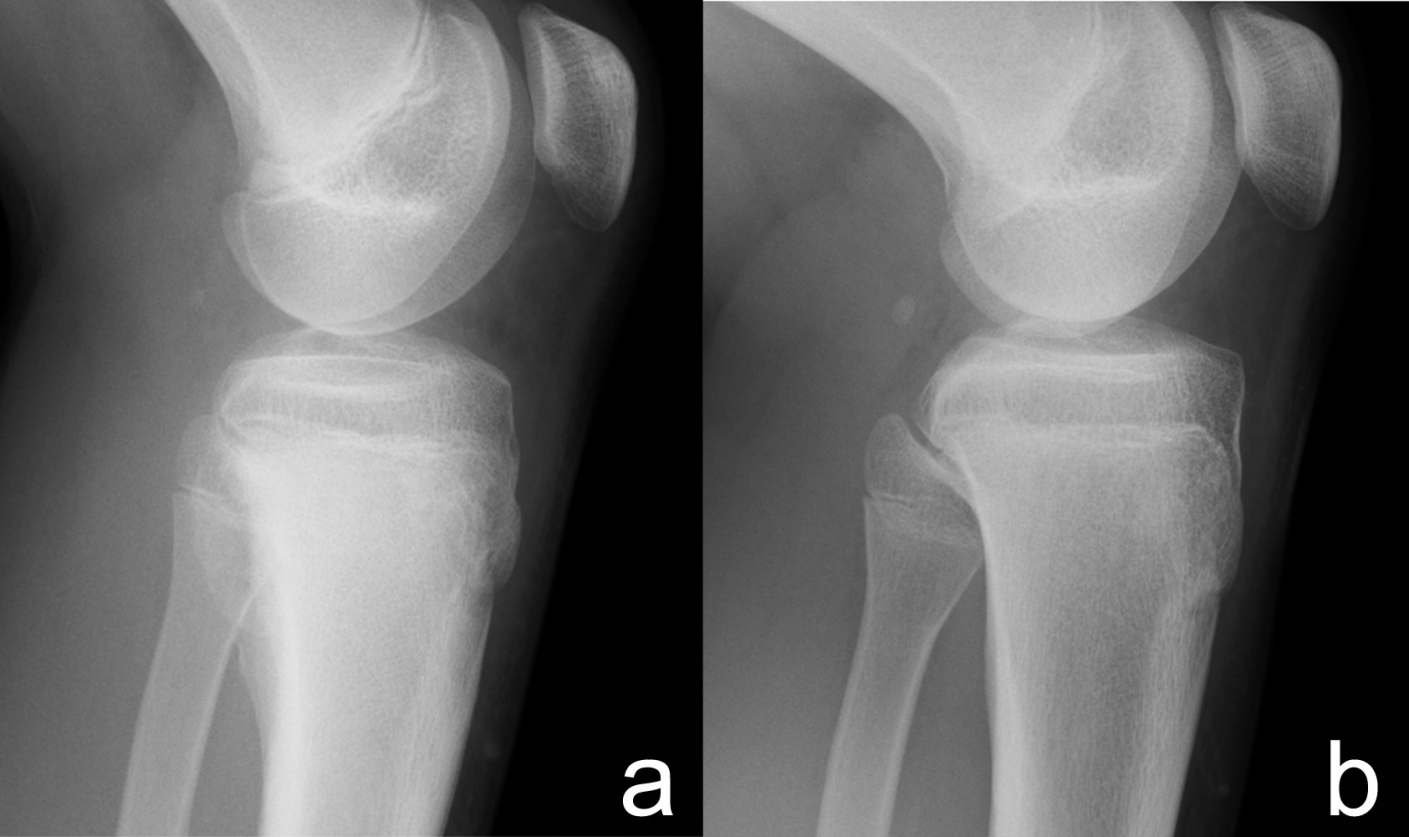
X-ray findings of the lesion. Shell-like lesion on the posterior surface of the tibia before operation (a) completely disappeared and the host bone had consolidated well 2 years after operation (b).
